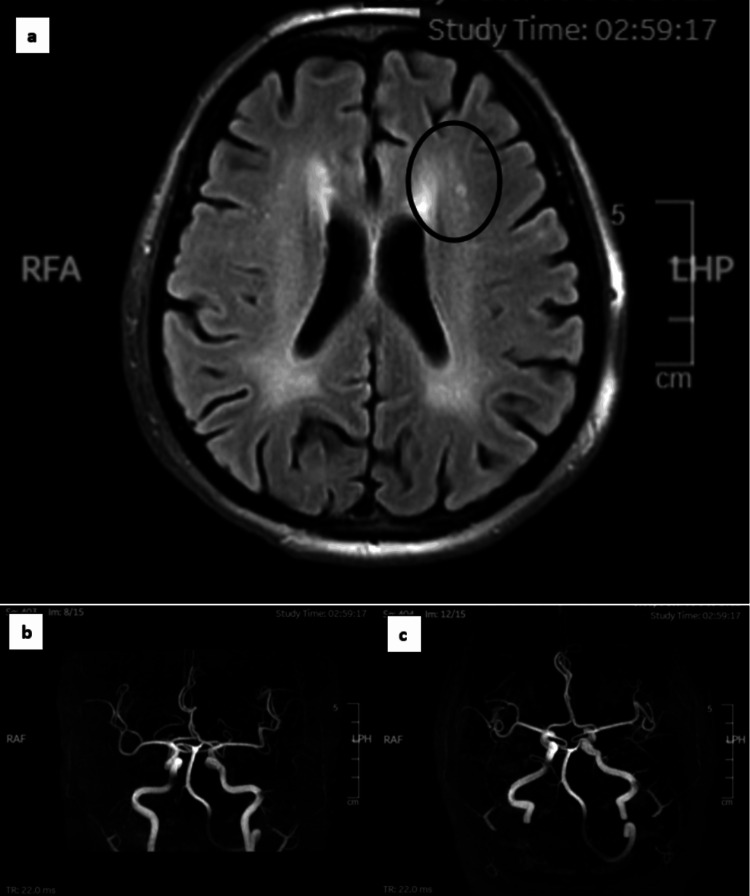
Neuro-imaging in the acute setting. a) MRI of the brain (axial DWI) reveals a small (<15mm in diameter) and deep left lacunar infarction (depicted by the black circle). b) Magnetic resonance angiography (MRA) of the Circle of Willis (anteroposterior view) demonstrates the continuous structure of the Circle of Willis, with no stenosis observed along the basilar artery, middle cerebral arteries, and penetrating arteries. c) Magnetic resonance angiography (MRA) of the Circle of Willis (infero-superior view) provides a different perspective, confirming the continuity of the Circle of Willis, with no evident stenosis changes observed along the basilar artery, middle cerebral arteries, and penetrating arteries.
